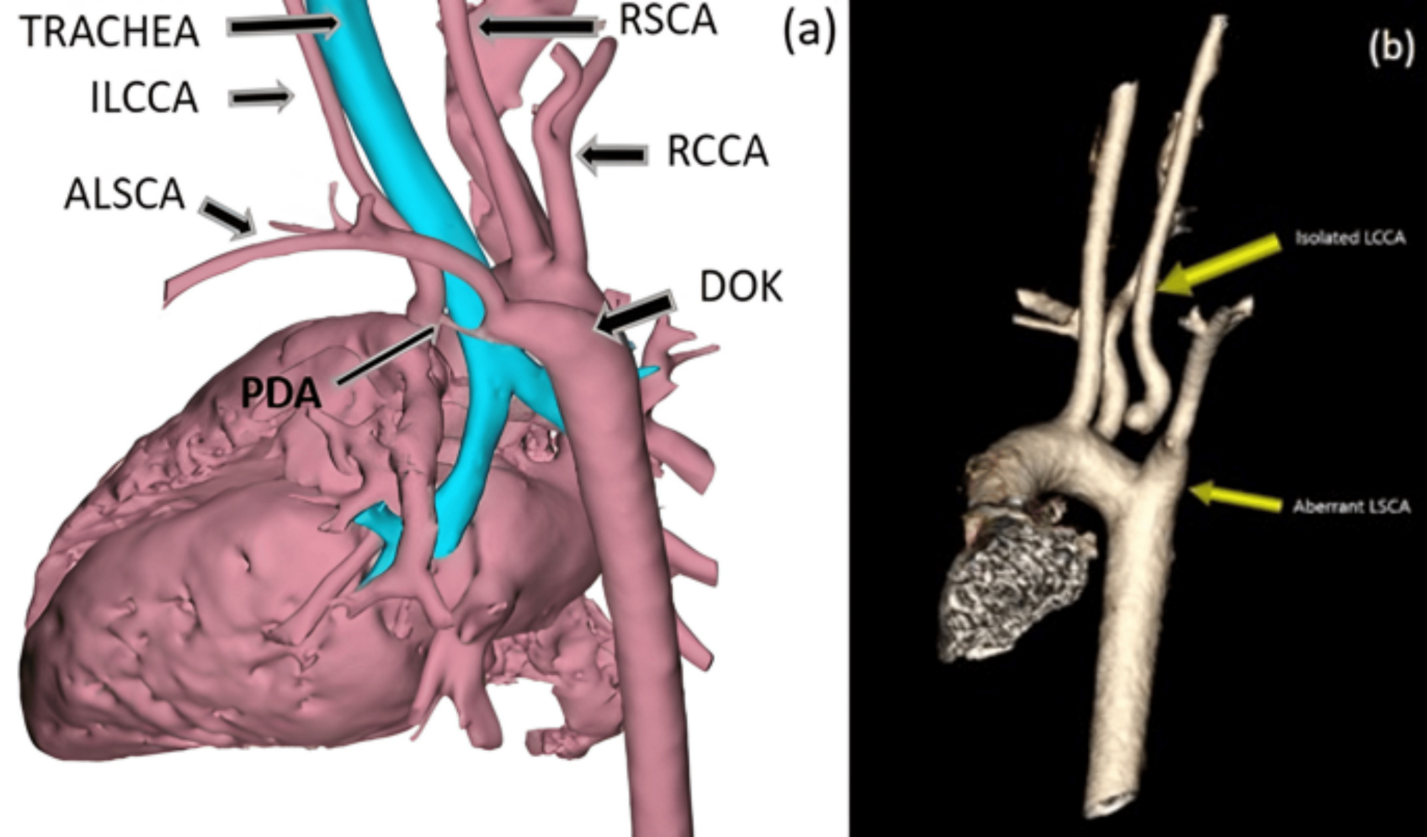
Annotated 3D models generated from the CT angiogram (a) 3D modelling showing the aortic arch branching and vascular ring circling the trachea. (b) 3D rendering of the aortic arch vessels showing the isolated LCCA and aberrant LSCA. ALSCA: Aberrant left subclavian artery; DOK: Diverticulum of Kommerell; ILCCA: Isolated left common carotid artery; PDA: Patent ductus arteriosus; RCCA: Right common carotid artery; RSCA: Right subclavian artery
